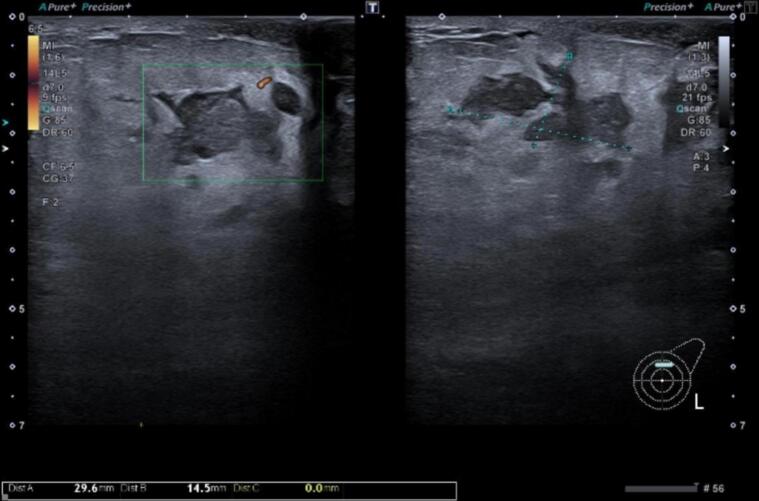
Ultrasound of left breast shows interval development of increase in skin thickness and parenchymal fuzziness with minimal edema and increased echogenicity especially in the *peri* areolar region with tenderness. Interval development of a few new ductal segments with internal non-homogenous avascular echoes.
